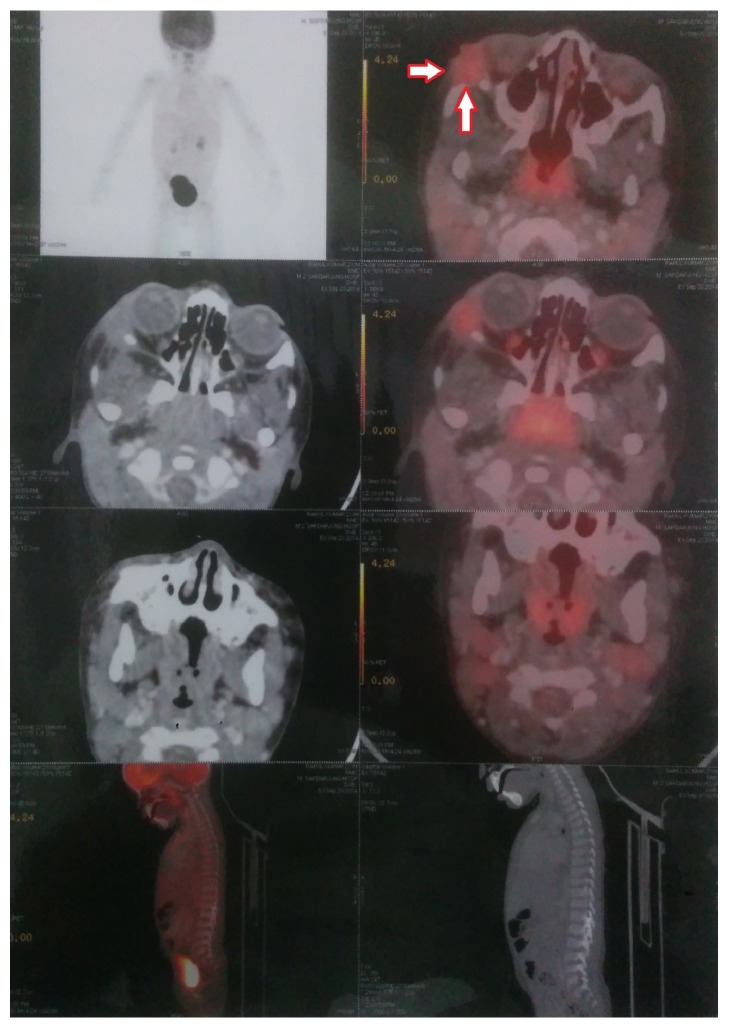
PET-CT scan with arrows marked showing increased uptake suggestive of increased activity at the site of the eosinophilic granuloma (Langerhans Cell Histiocytosis).
